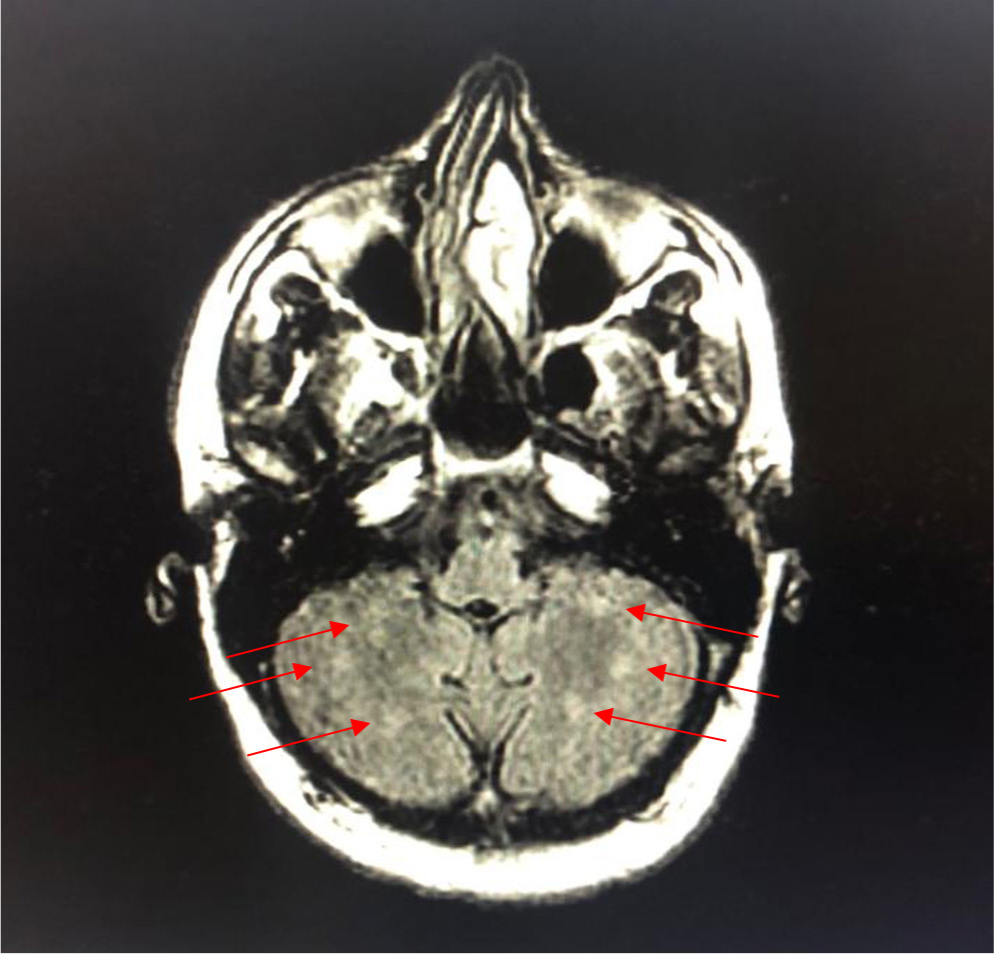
T2 FLAIR MRI of the brain in August year X showing multiple nodular signal abnormalities of the cerebellar hemispheres.
